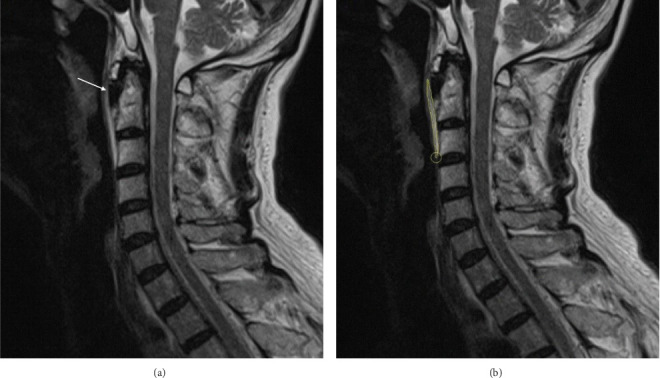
(a) Cervical MRI shows calcification of the longus colli muscle at the level of C1 and C2 (arrow heads). (b) ROIs are delineated to outline the extent of the lesion (edema in the prevertebral soft tissues). ROIs, regions of interest.
